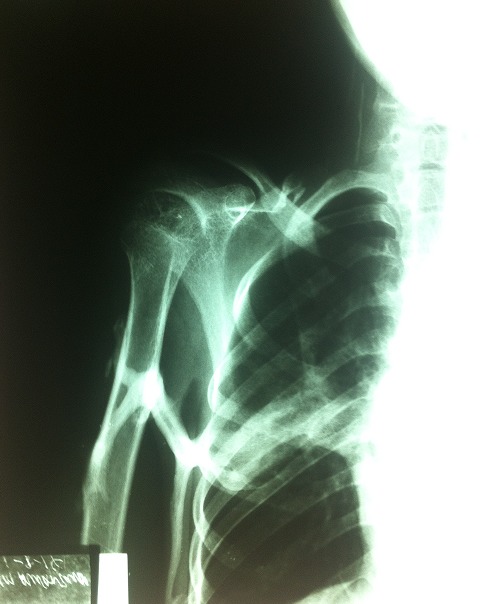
Radiograph, showing the hallux valgus of the right great toe
